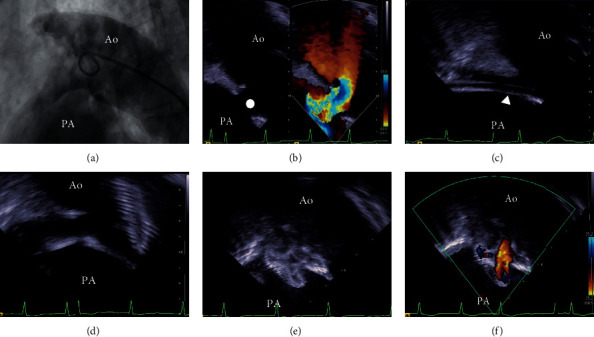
Representative case where ICE is a valuable guide for TC-PDA. Patient 23 has a large Krichenko type B PDA that was not delineated by an aortography (a) but was clearly visualized by ICE (closed circle) with a 11.1 mm PA side diameter and a 3.9 mm length (b). We chose Amplatzer septal occluder 17 mm for the closure of this PDA. During TC-PDA, ICE showed all process of procedure. We advanced a long sheath (arrowhead) through the PDA (c), opened the aortic skirt in the aorta (d), and pulled the entire system back and deployed right atrial disc (e). Finally, ICE showed small residual shunt just beside the body of device (f) and we finished the procedure successfully. Ao, aorta; ICE, intracardiac echocardiography; PA, pulmonary artery; PDA, patent ductus arteriosus; TC- PDA, transcatheter closure of patent ductus arteriosus.
